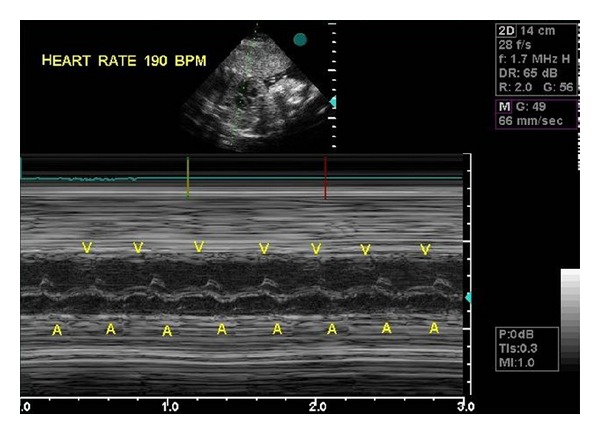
M-mode fetal echocardiogram shows sinus tachycardia of 180–190 per minute.
